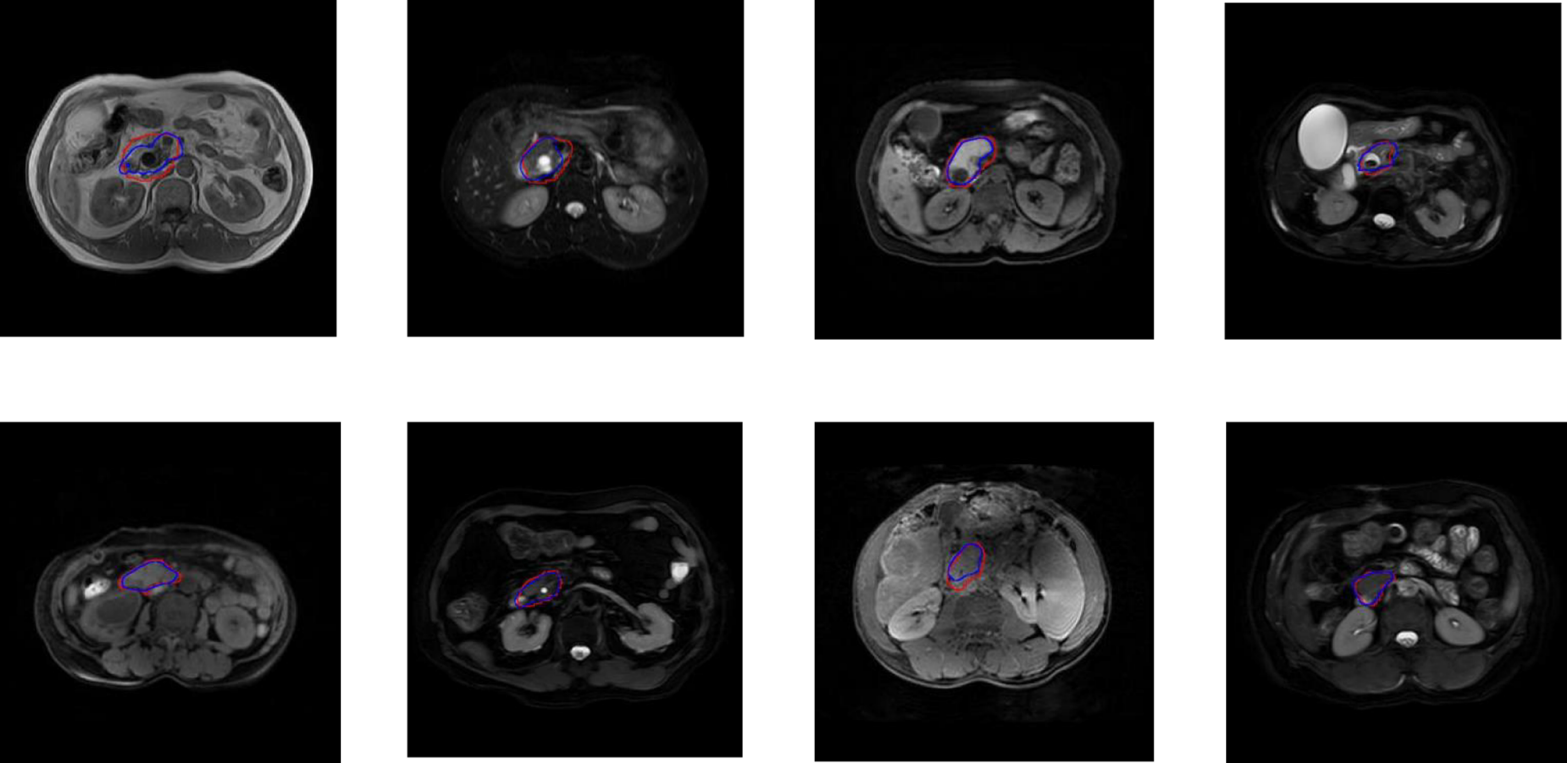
Examples of PA regions of PAC patients (top panel) and PA regions of patients without PAC (bottom panel). The first column were examples of T1 MRI image obtained by UNet16 trained using only T1 images, the second column were examples of T2 MRI image obtained by FCNRes50 trained using only T2 images, the third column were examples of T1 MRI image obtained by UNet16 trained using both T1 and T2 images, and the fourth column were examples of T2 MRI image obtained by UNet16 trained using both T1 and T2 images. Blue, algorithm; red, expert.
